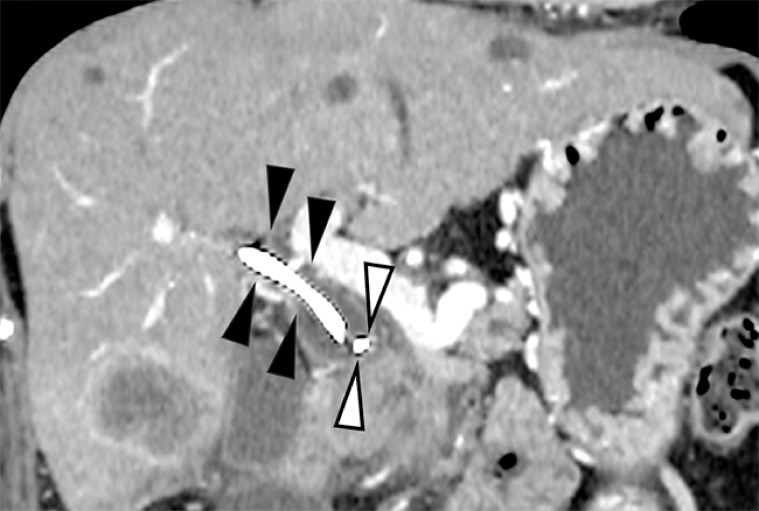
Contrast-enhanced computed tomography revealed migration of a plastic stent (black arrowheads), with the distal end located above the stricture in the distal common bile duct. The proximal flap of the plastic stent is also seen (white arrowheads).
